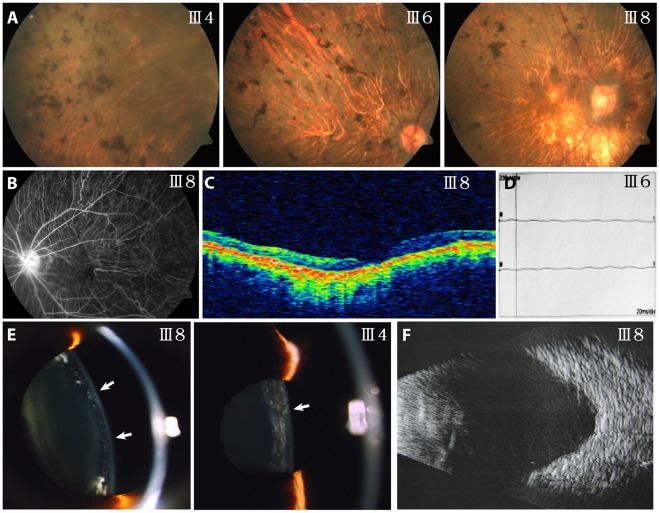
Representative photographs of patients of this family. (A) Fundus photographs showing bone spicule-like pigmentation, optic never head epimembrane and retinal vascular attenuation. Chorioretinal degeneration with peripapillary atrophy was seen. (B) Fundus fluorescein angiography images showing retinal vascular attenuation and chorioretinal atrophy. (C) OCT showing retinal atrophy. (D) ERG records showing no detectable cone and rod responses. (E) Slit-lamp photography showing punctate opacity of the lens as indicted by the arrows. (F) B-scan ophthalmic ultrasonic images showing posterior scleral staphyloma, indicating high myopia.
